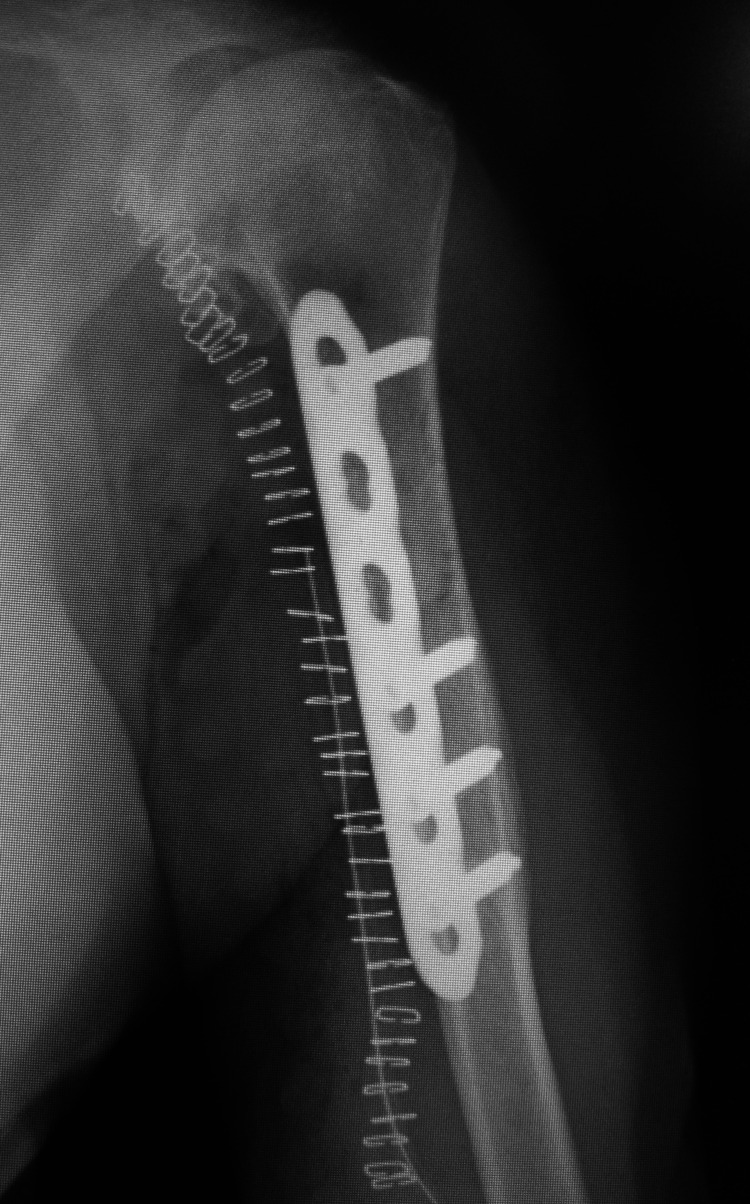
Postoperative antero-posterior radiograph of the left upper arm showing the fragment of cortical bone fixed with a plate.
